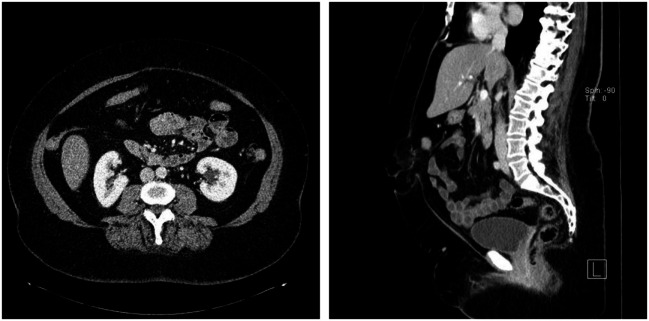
CT scan of the patient with rectus abdominis diastasis, and umbilical and epigastric hernia.
